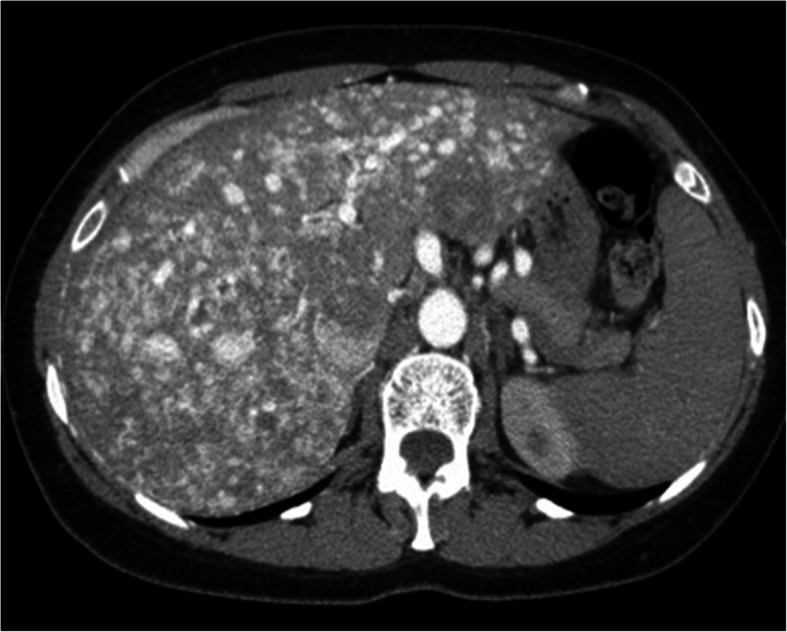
Axial CT with contrast of a 45-year-old woman with HHT demonstrates innumerable enhancing arteriovenous malformations throughout the liver parenchyma. Partially visualized is hypertrophy of the celiac artery
